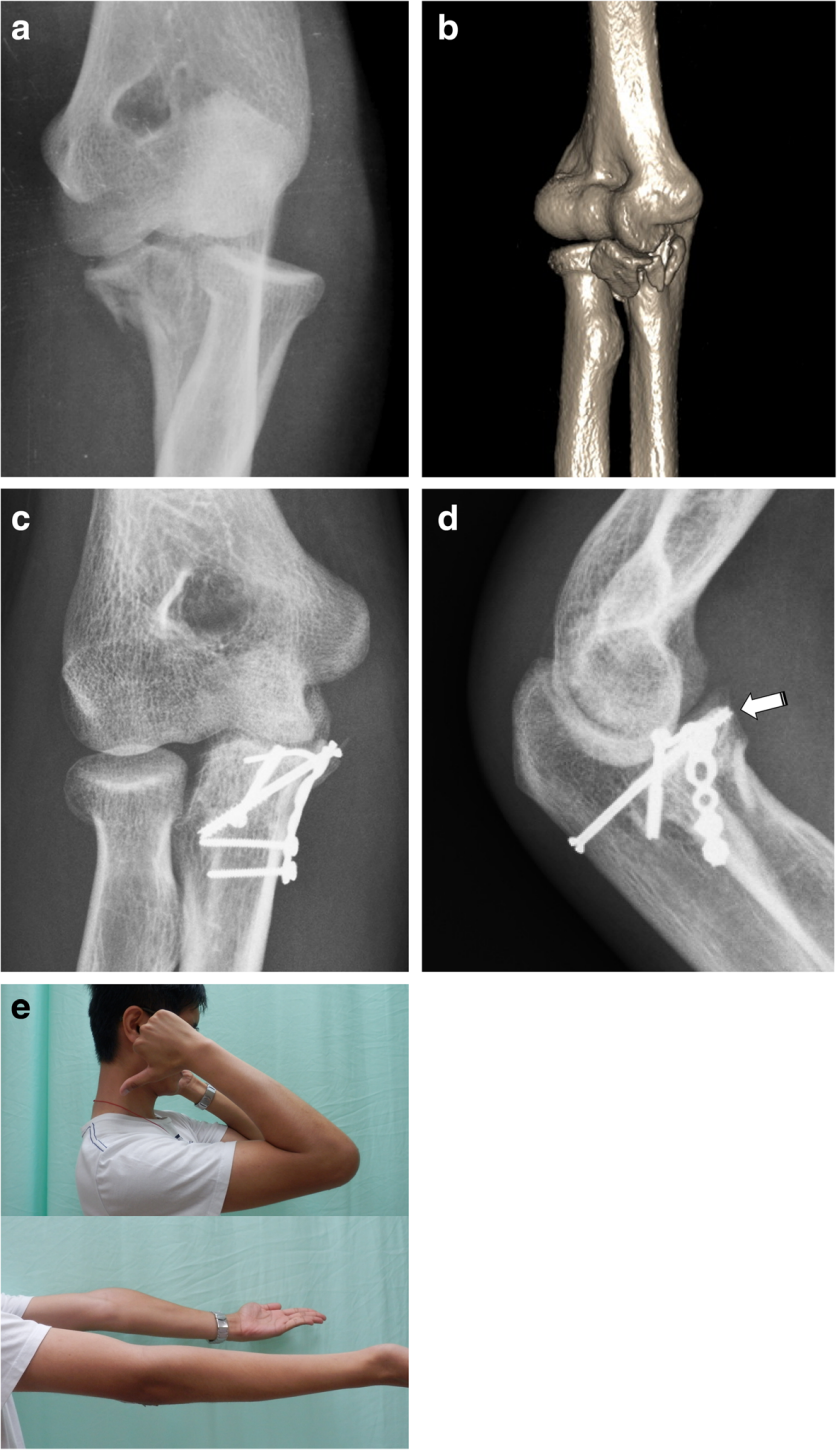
Anteromedial facet fracture with varus instability. a Plain radiograph. b Three-dimensional computed tomography image showing a large coronoid fragment. c Postoperative anteroposterior radiograph. d Postoperative lateral radiograph; the white arrow shows retrograde screw fixation of a coronoid base fracture. e Patient photo at 10 weeks after surgery showing good range of motion
